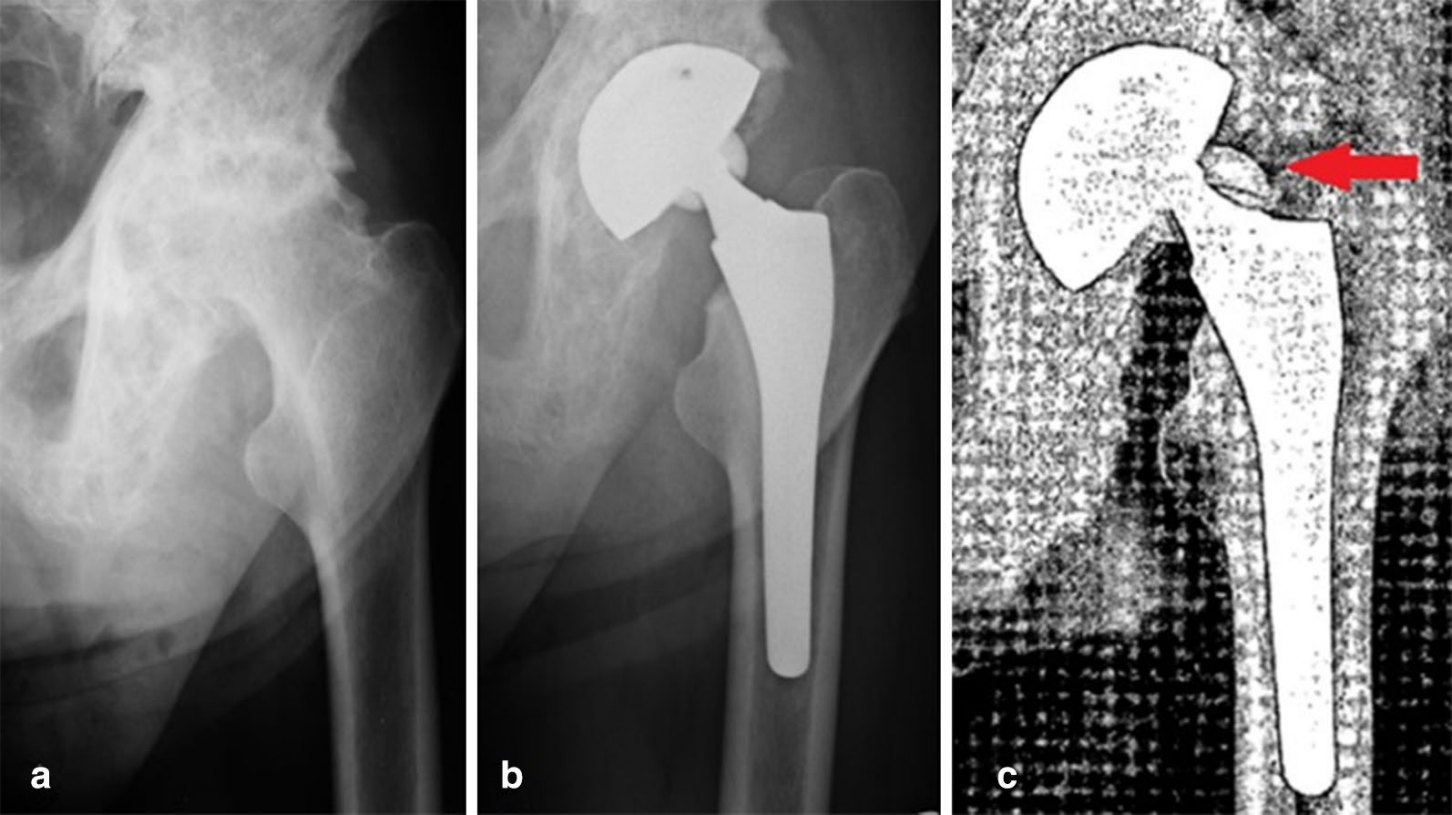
a Pre-operative anteroposterior X-ray of the left hip of a man who was 76 years old at the time of surgery, showing arthrosis secondary to Paget’s disease affecting the upper femur; b post-operative radiograph: cementless THA; c anteroposterior view of the pelvis after traumatic ceramic head fracture occurred 64 months after surgery
